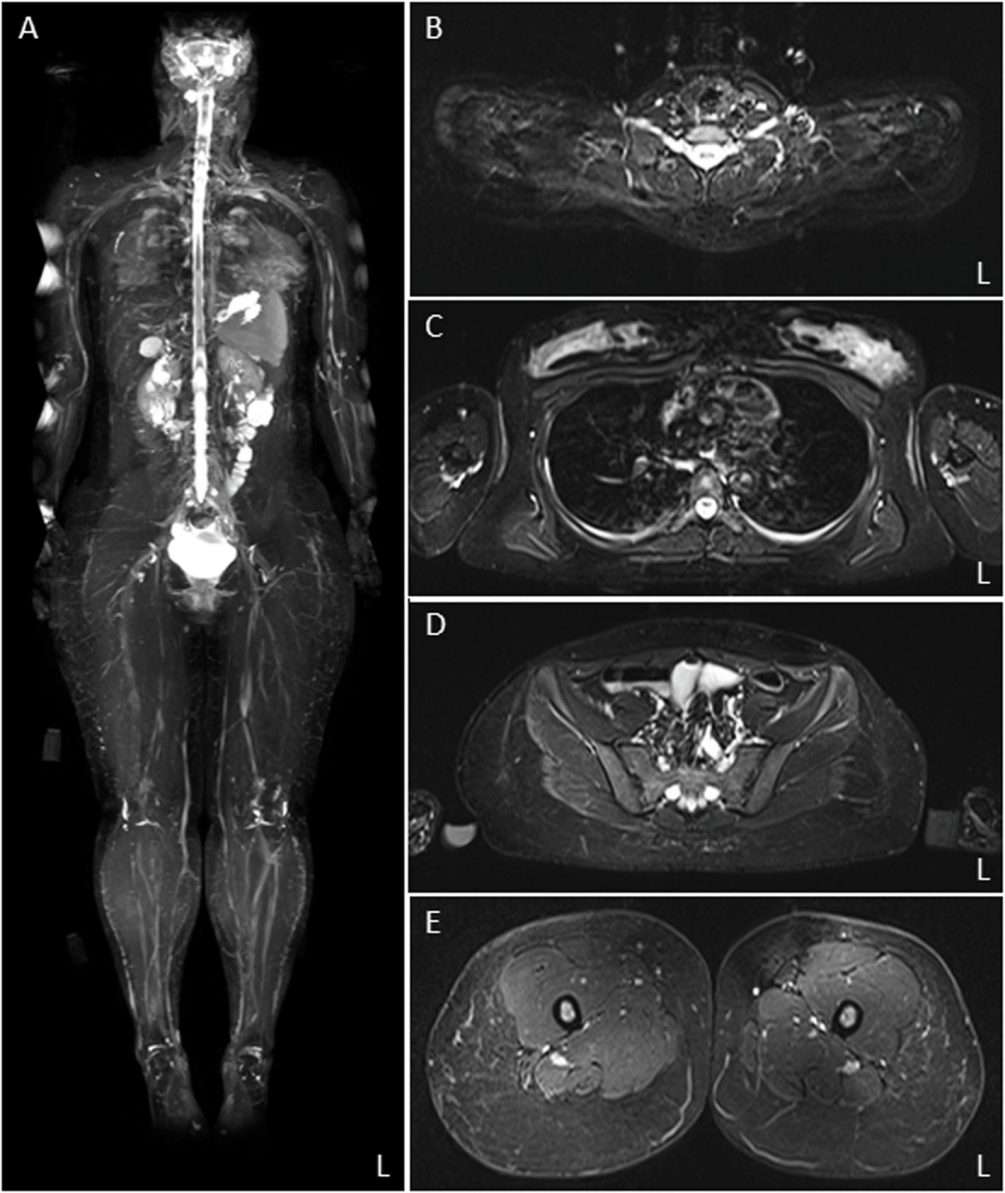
Magnetic resonance imaging with short tau inversion recovery sequences in the proband’s sister with CMT1A. Coronal magnetic resonance neurography (A), and axial images (B-E) do not reveal an extreme nerve thickening, as observed in the proband
